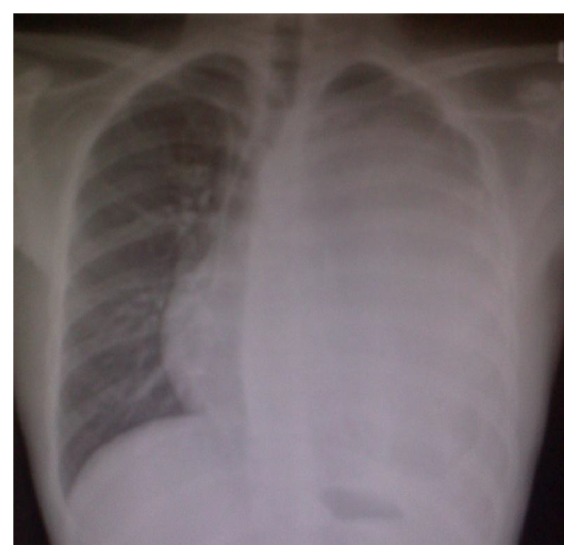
Chest radiograph showing left mediastinum mass.
